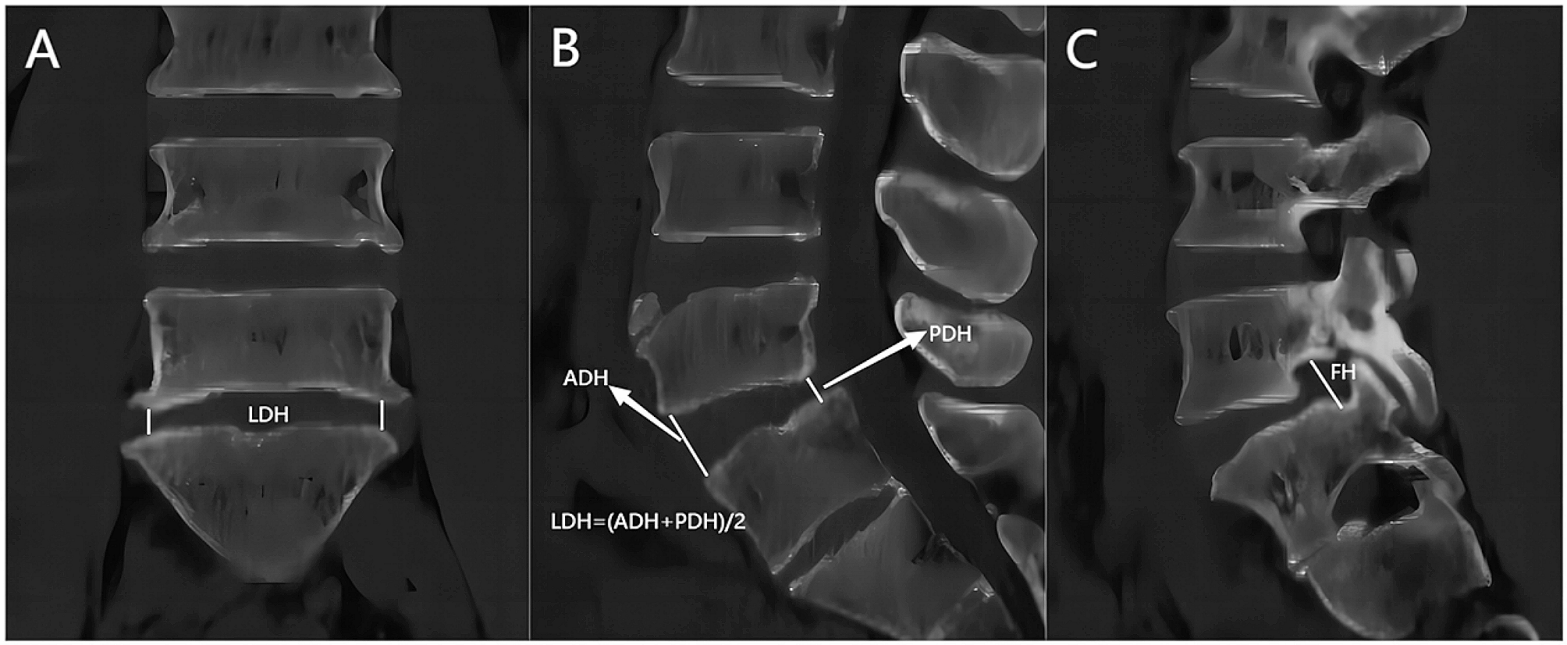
(A) The lateral disc height (LDH) was the lateral marginal height of midline intervertebral space of CT coronal vertebrae. (B) The disc height (DH) was the average of the disc height(ADH) and posterior disc height(PDH) in the sagittal CT. (C) The foraminal height (FH) was the distance between the inferior edge of the pedicle of the upper vertebral body of CT and the upper edge of the pedicle of the lower vertebral body
